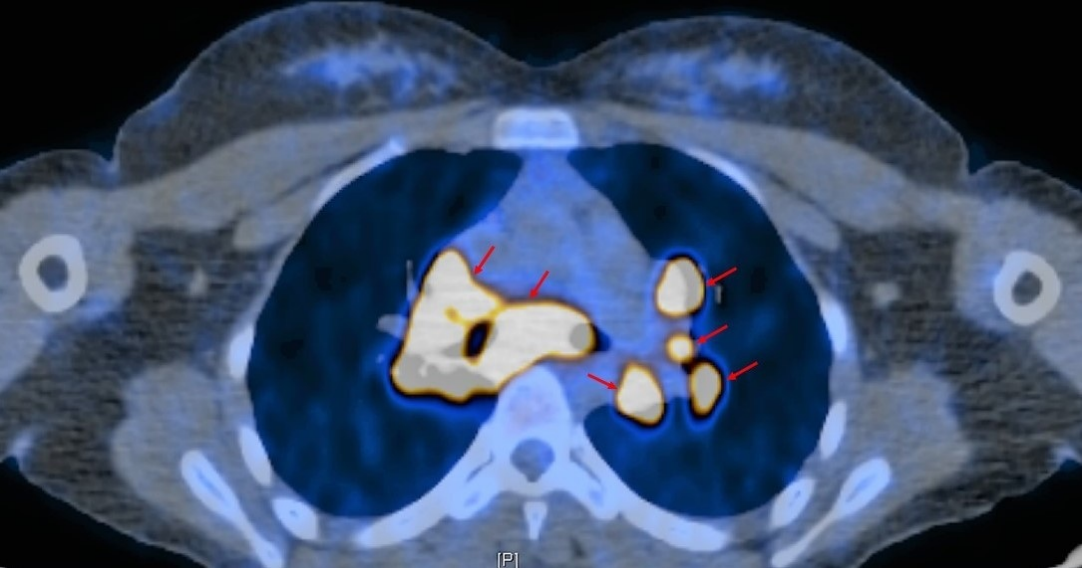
High metabolic activity on positron emission tomography/computed tomography (PET/CT) of the chest is concerning for metastatic disease
